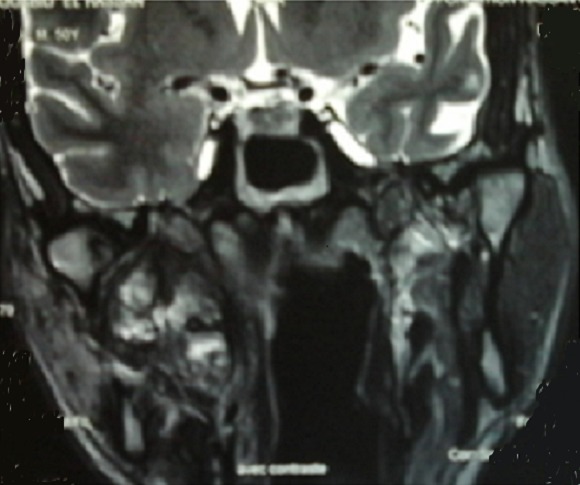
MRI appearance 20 months after radiotherapy
